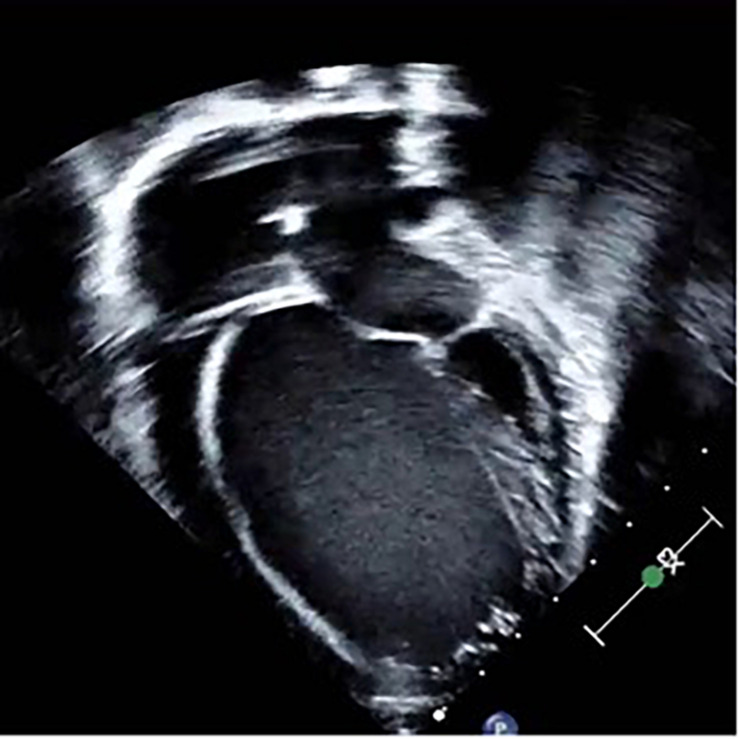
Two-dimensional, apical 4-chamber echocardiographic image depicting an enlarged ventricle with spherical geometry and bilateral atrial enlargement in a patient harboring dilated cardiomyopathy secondary to a pathogenic gene variant in the *TTN* gene.
